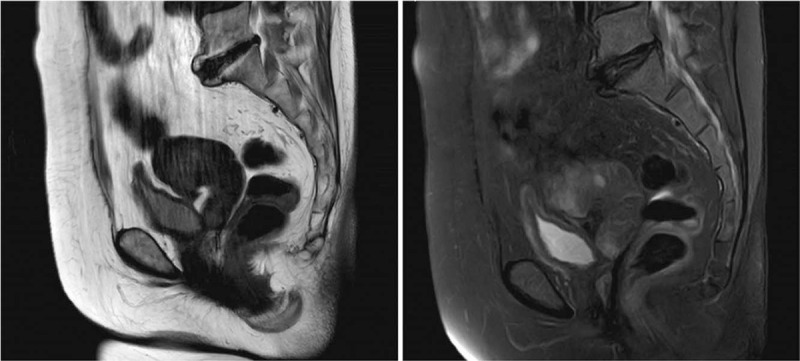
A pelvic MRI showing absence of the uterus, and closure of the os of the vagina, forming a closed loop full of fluid.
